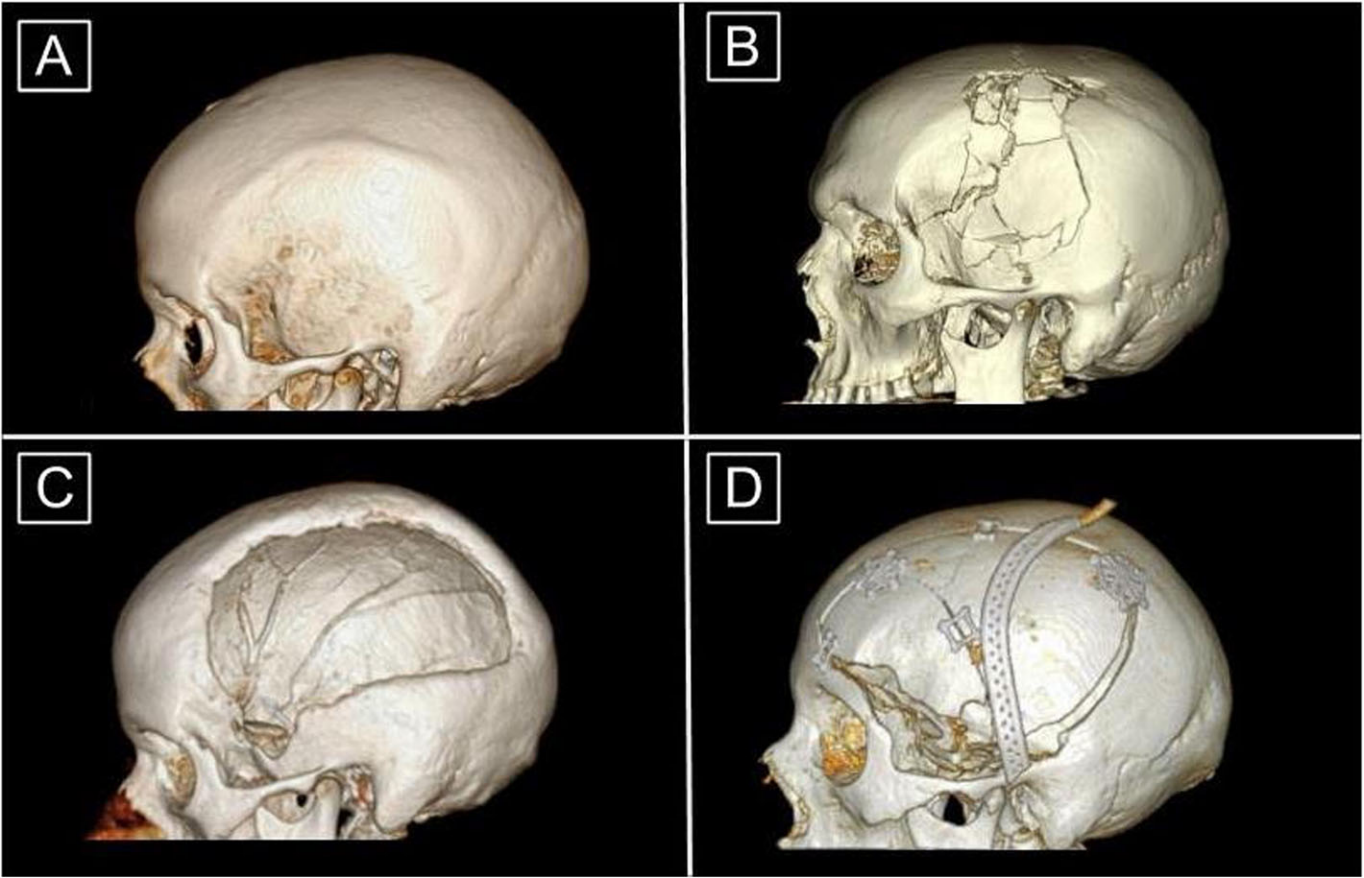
3D Reconstructions of Head CT Images in Craniectomy and Cranioplasty (a) Intact or normal skull. (b) Skull fracture that is overlying intracranial pathology requiring craniectomy. (c) Cranial defect after craniectomy. Note the asymmetric and heterogeneous nature of the outer border. (d) Post-operative subject who had undergone cranioplasty with autologous bone. The perforated piece overlying the skull is a Jackson-Pratt drain. Note that the bone fragments require extra structural support and do not fully repair the cranial defect along the inferior border
